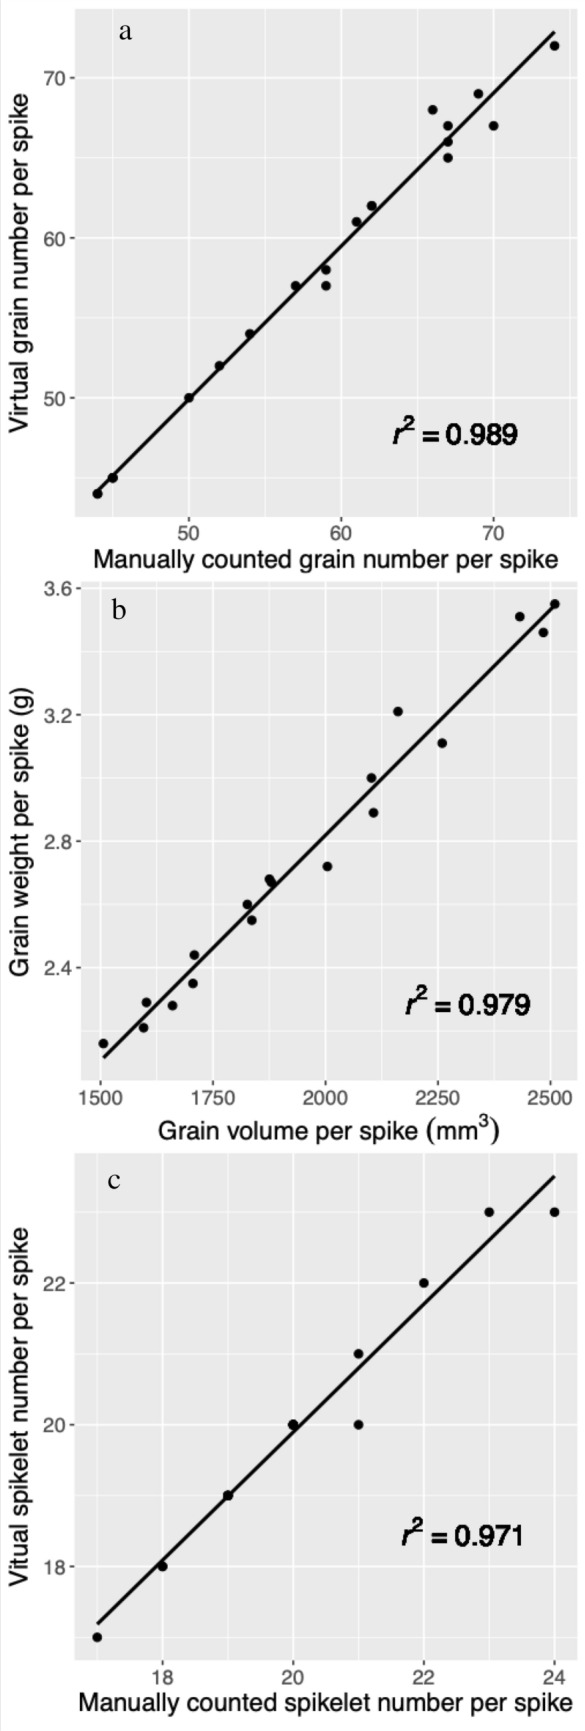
Validation of the CT imaging-based method using a manual counting-based method. a grain number, b relationship between volume and mass of grains per spike, c spikelet number
